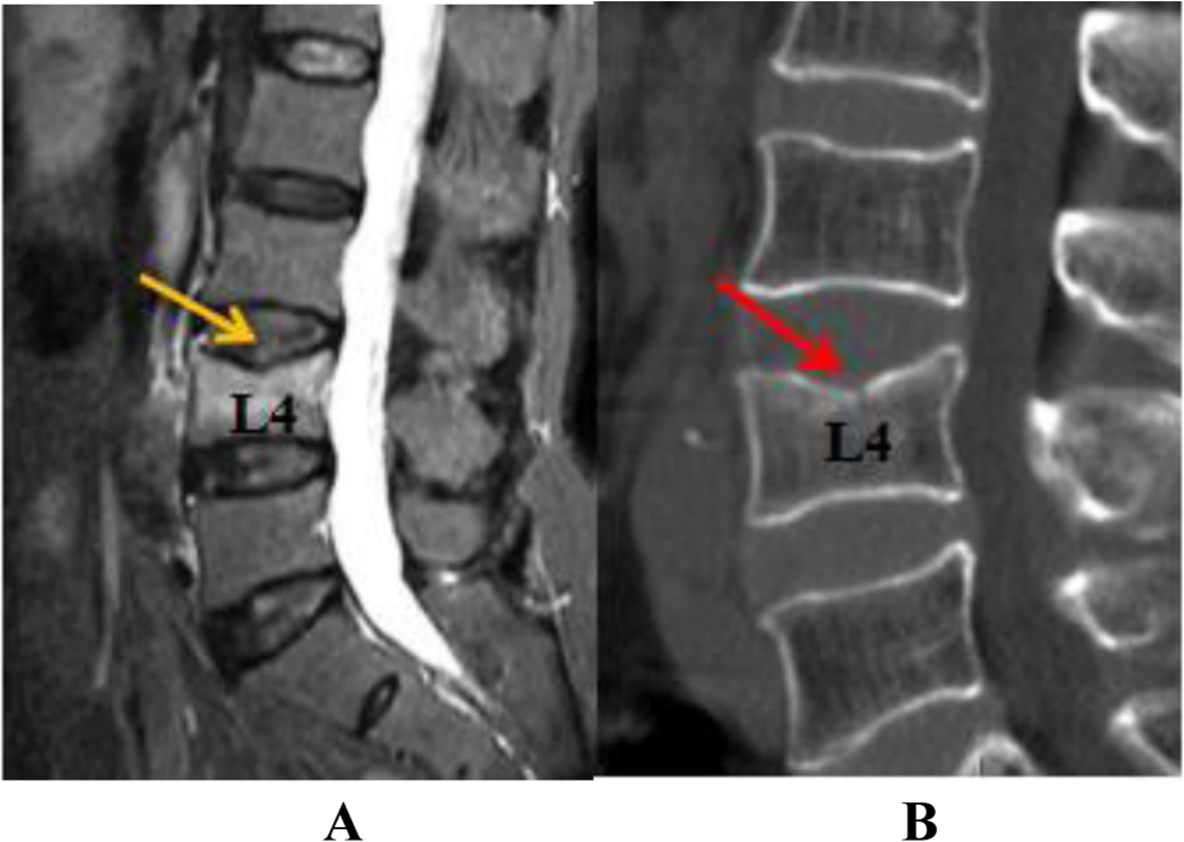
A 76-year-old woman with OVF at level L4. Panel a is an MRI STIR image. The yellow arrow indicates a diffuse high signal in the OVF on the cranial side of the fractured vertebral body and a fracture of the upper endplate of the vertebral body. Panel b is a CT image of the fractured vertebral body. The red arrow indicates a fracture of the upper endplate of the L4 vertebral body. The endplate has collapsed with signs of bone sclerosis below the endplate. This is a grade 3 EDC injury as delineated in this study
